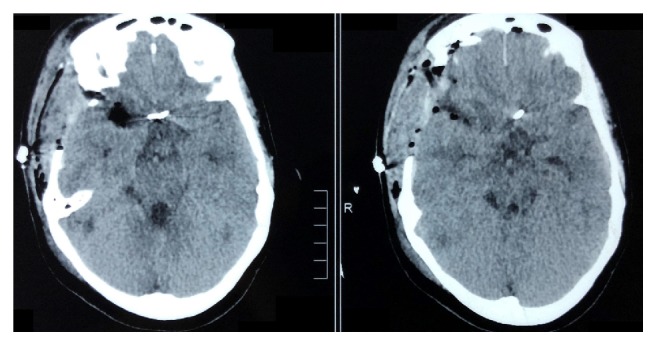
Postoperative plain Computed Tomogram scan of the brain showing the absence of hematoma or infarct.
